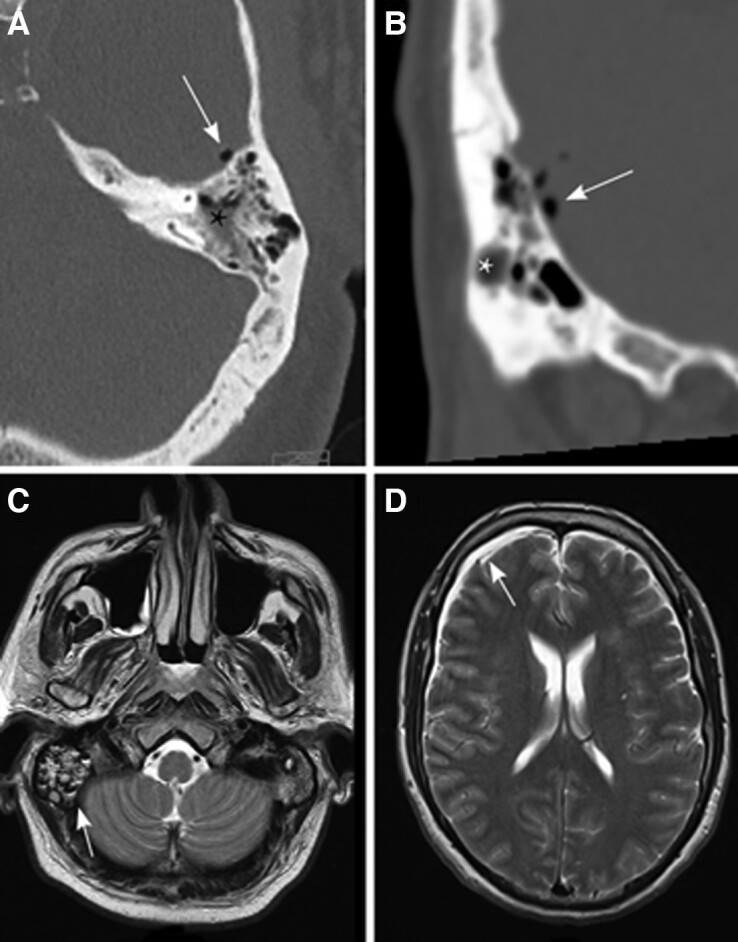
Cranial imaging of patients with otogenic meningitis. Axial (*A*) and coronal (*B*) computed tomography showing mastoid opacification (asterisk) and intracranial air (arrow). Axial T2 weighed cranial MRI showing opacification of the right mastoid in a meningitis patients (*C*, arrow), and hyperintense subdural fluid collection consistent with empyema (*D*). Abbreviation: MRI, magnetic resonance imaging.
